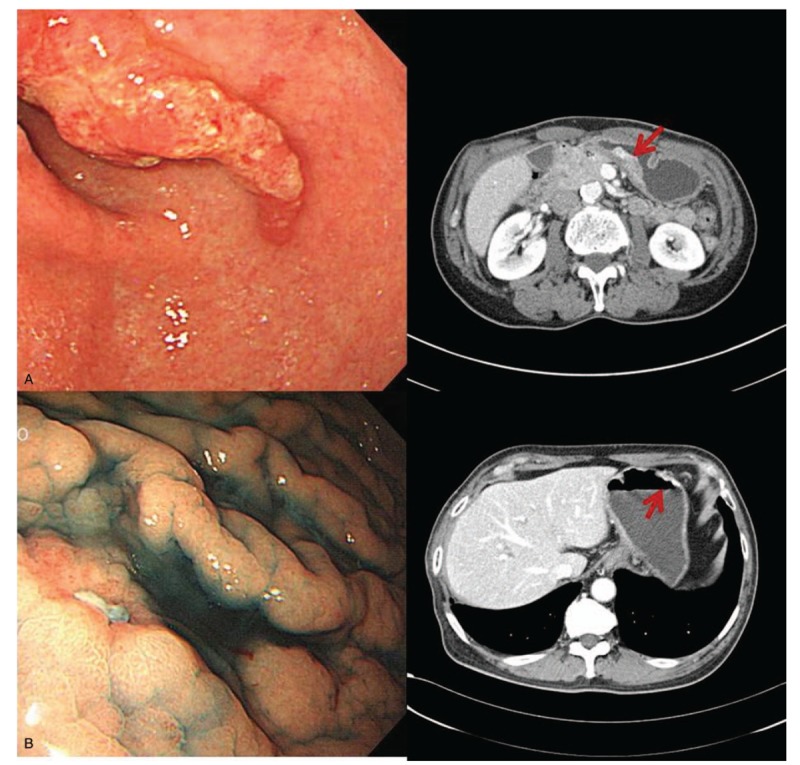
(A) 71-years-old-male patient. He had EGC with deep SM invasion at antrum. Elevated lesion was found in gastroscopy and polypoid lesion was also found in CT scan. (B) 68-years-old -male patient. Central depressed lesion was found in gastroscopy and CT scan.
